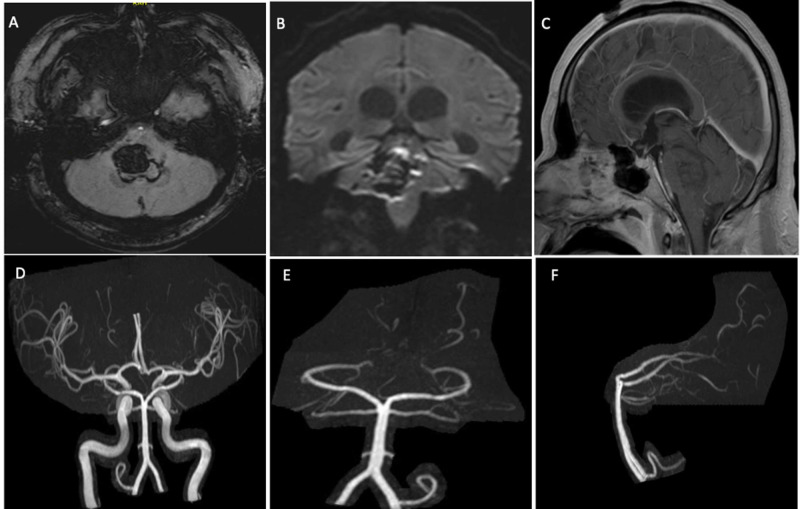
Brain MRI with and without contrast showing brainstem hematoma centered in the pons, just to the right of midline with extension into the right middle cerebral peduncle associated with intraventricular extension (A) Axial susceptibility-weighted image at the level of the pons showing the brainstem hematoma (B) Coronal diffusion-weighted image at the level of the brainstem showing the largest diameter of intraparenchymal hematoma (C) Sagittal post-gadolinium T1 image at the midline demonstrating the lack of contrast enhancement at the hematoma (D) Anteroposterior MRA reconstruction showing both the anterior and posterior circulations demonstrating a normal circle of Willis (E) Anteroposterior MRA reconstruction showing the posterior circulation with no evidence of arteriovenous malformation (F) Lateral MRA reconstruction showing the posterior circulation with no evidence of arteriovenous malformation
